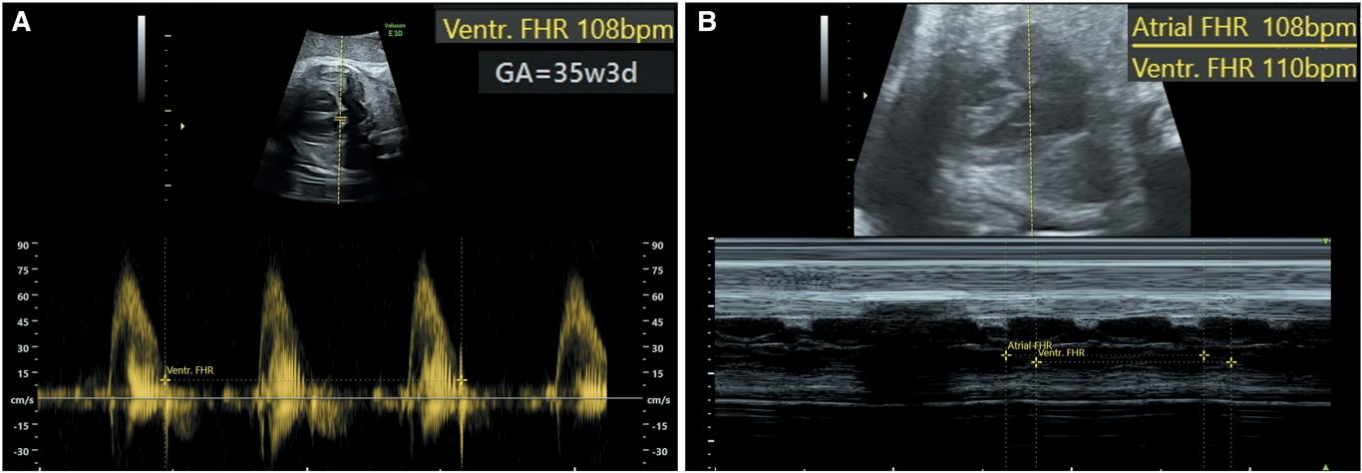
Foetal echocardiography at 35-week gestation showing a ventricular heart rate of 108 b.p.m. (*A*) with normal atrioventricular intervals (*B*).
